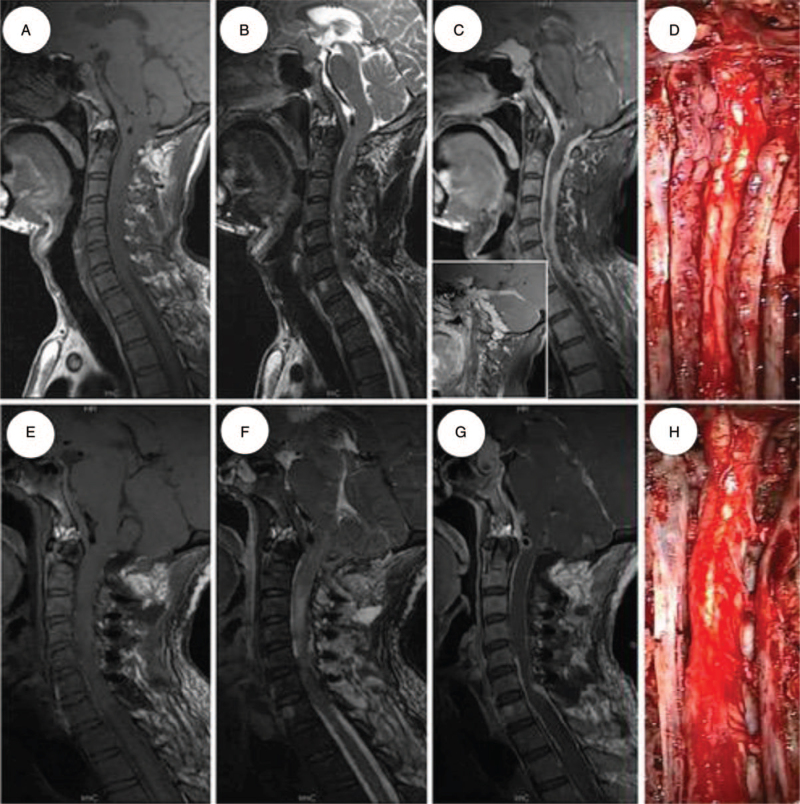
Preoperative (A-C) magnetic resonance image (MRI) shows the lesion extends from tentorium cerebella to the sixth cervical spinal canal and enveloping the cervical spinal cord with isointense on T1-weighted image (T1WI) and T2-weighted image (T2WI)(A-B), and enhanced remarkably and homogeneously after infusion of Gd-DTPA (C), and postoperative (E-G) MRI revealed the tumor in the cervical spinal canal was almost resected, without excision of the lesion crawl on the tentorium cerebella and basis crania. Intraoperative photo (D, H): The tumor grew along the meninges displayed an irregular shape but clear boundaries with the spinal cord. The color of the tumor was greyish white with more than usually vascular, the cervical cord was compressed (D). After excision of the mass in the cervical canal, the spinal cord was decompressed, and cervical spinal cord roots are clear (H). Gd-DTPA = gadolinium-diethylene triamine pentaacetic acid.
